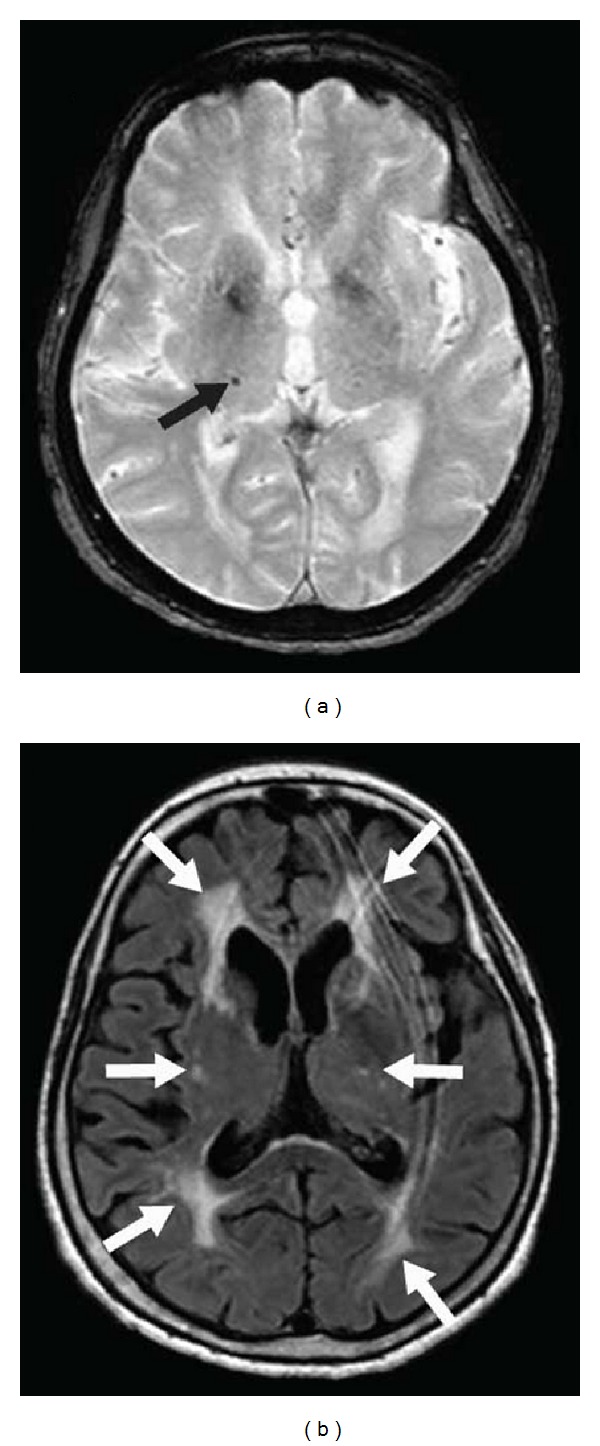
Gradient-echo (a) and FLAIR (b) sequences showing a right-sided thalamic microbleed (a) associated with diffuse ischemic white matter hyperintensities on FLAIR imaging (b) in a patient with severe and chronic arterial hypertension.
